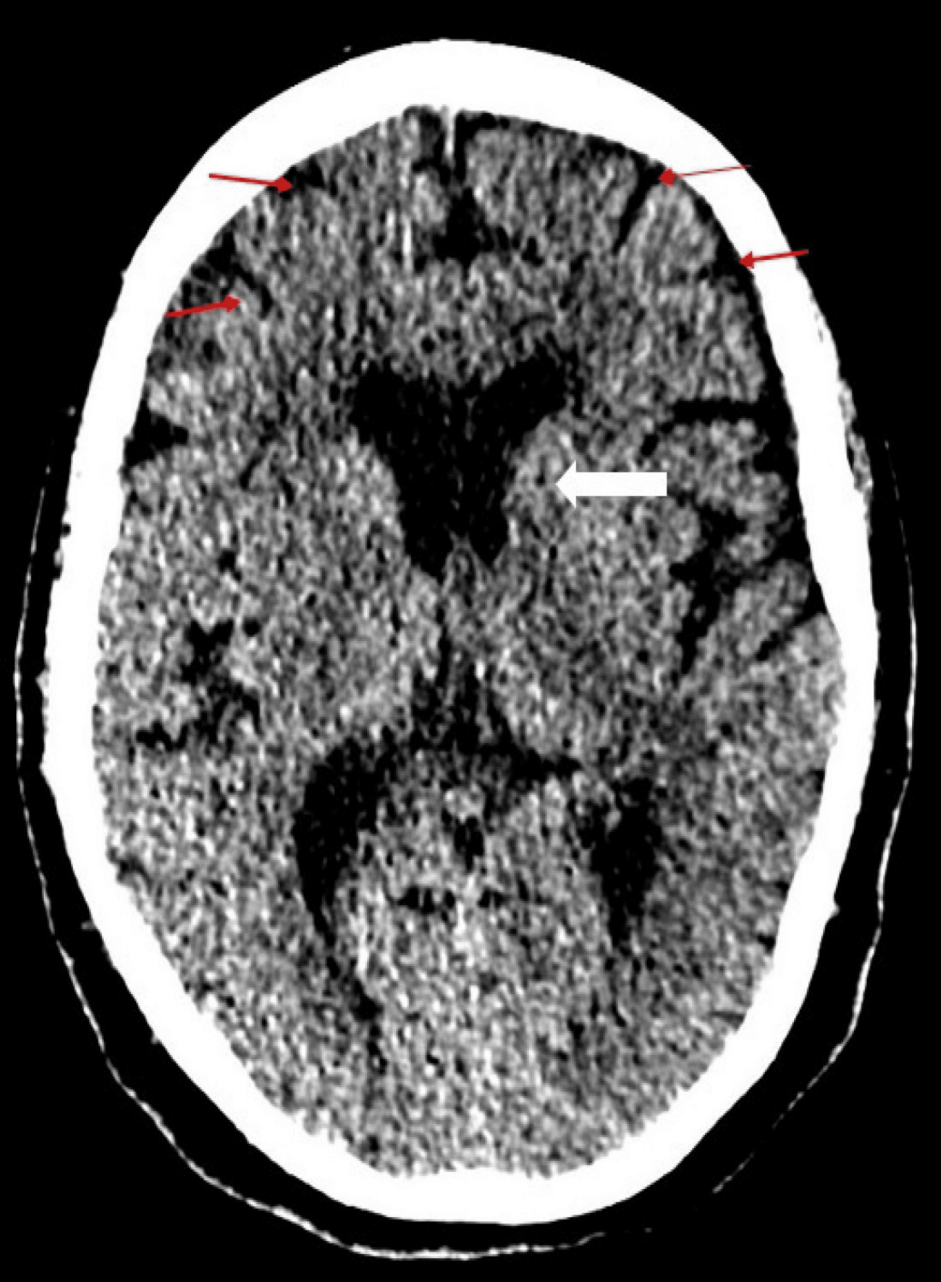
Axial computed tomogram (CT) head demonstrating a subtle old infarct in the left caudate nucleus (white arrow) and generalised brain involution signifying brain atrophy (red arrows).
